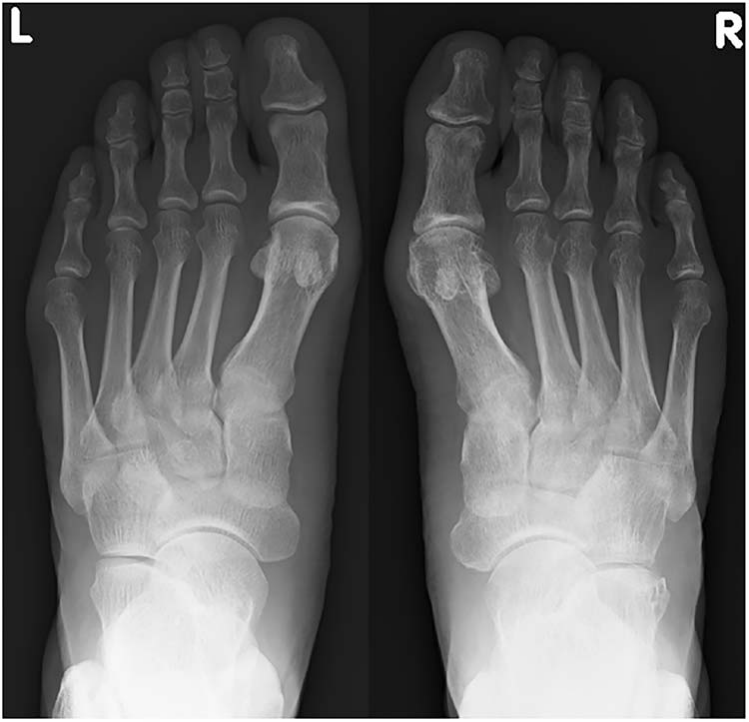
Plain radiograph at 1 year after the initial operation. There was no marked malalignment in the right foot.
